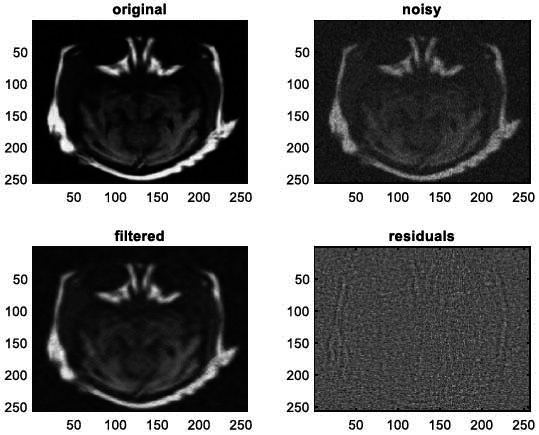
The twelfth example of MRI denoising by applying the proposed image denoising technique.
